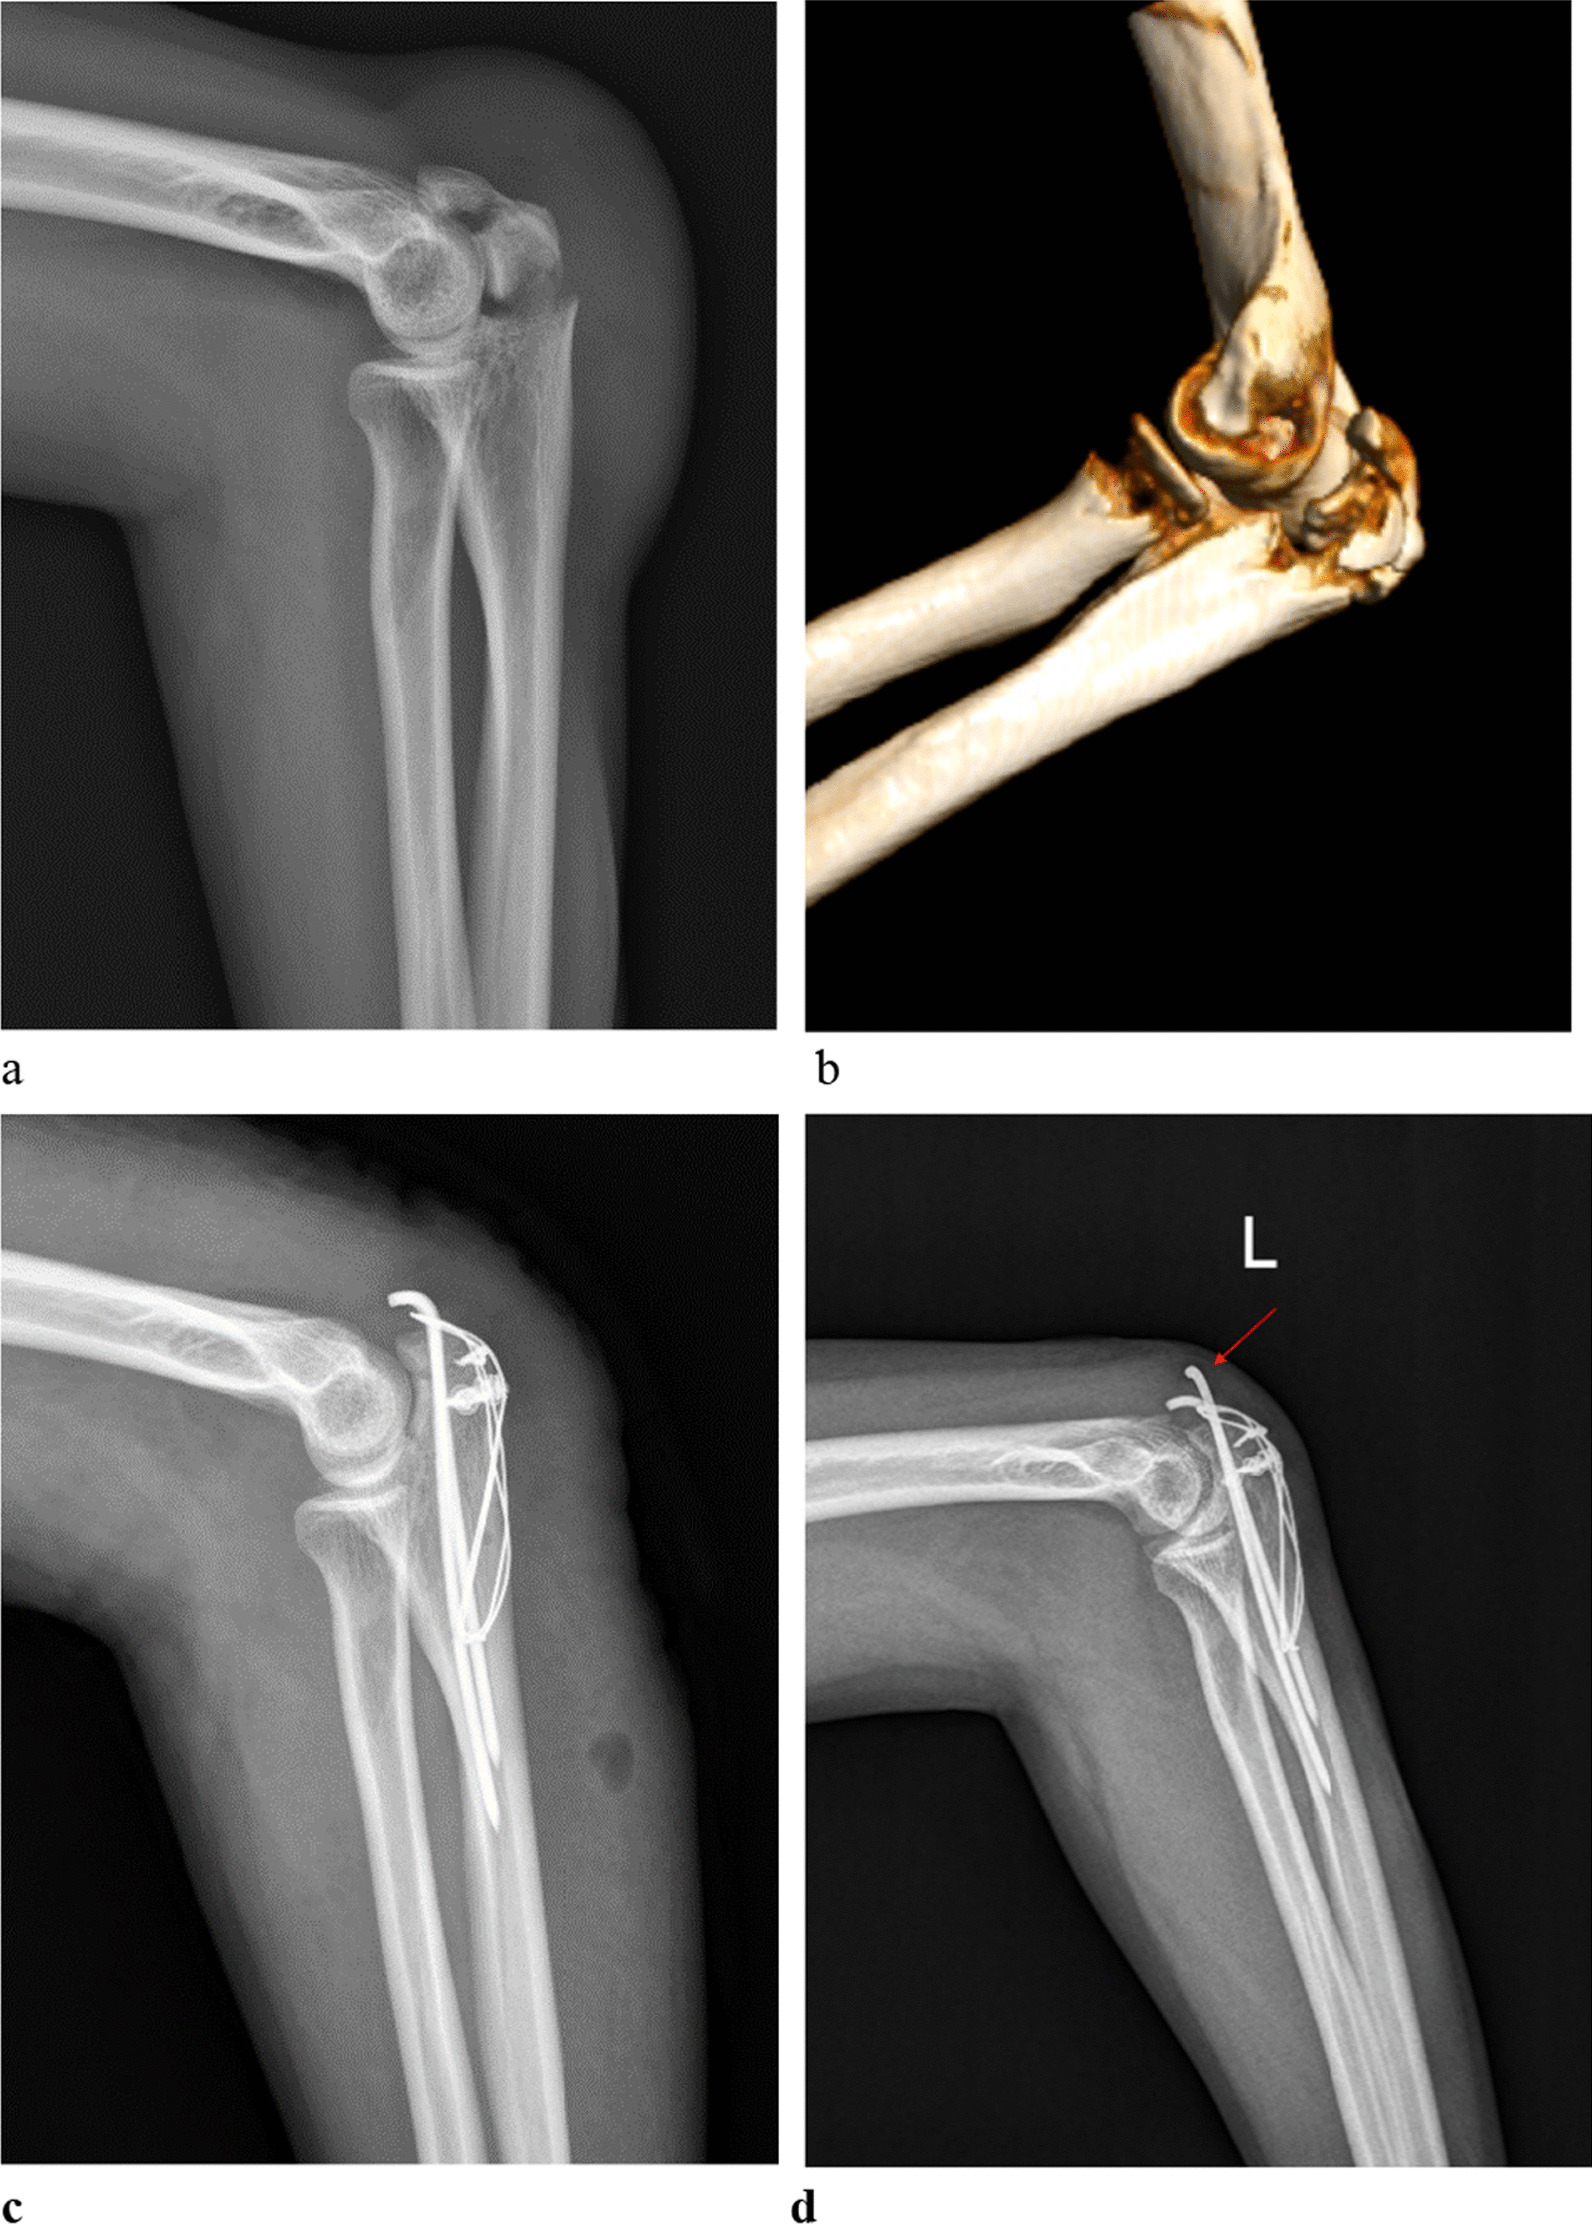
A 33-year-old man treated with free-hand TBW for olecranon fracture. Lateral-view radiograph (a) before surgery. Preoperative 3-dimensional reconstruction image (b). Immediate post-operative X-ray lateral view (c). Lateral-view radiograph at one-year follow-up showed K-wire backed out (d)
